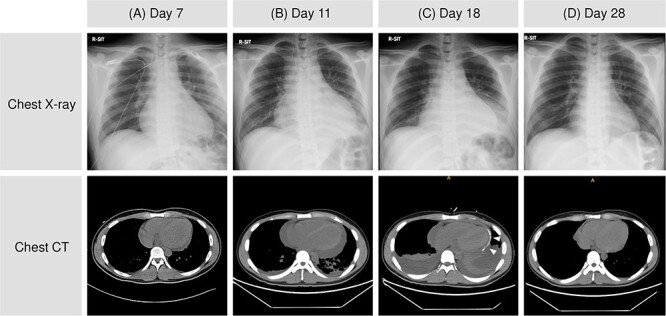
Chest X-ray and CT. Cardiac enlargement and pericardial effusion worsened (A and B) and pleural effusions became apparent after pericardial drainage (arrowheads) (C). Pericardial and pleural effusion then improved (D). CT: computed tomography.
